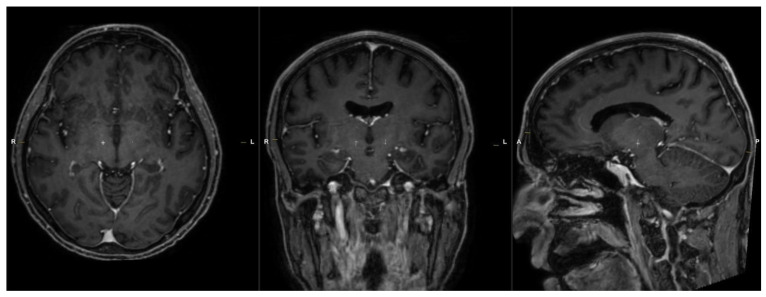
RebrAIn coordinates calculated on the same preoperative 3T MRI. The targets are indicated by the white cross.
